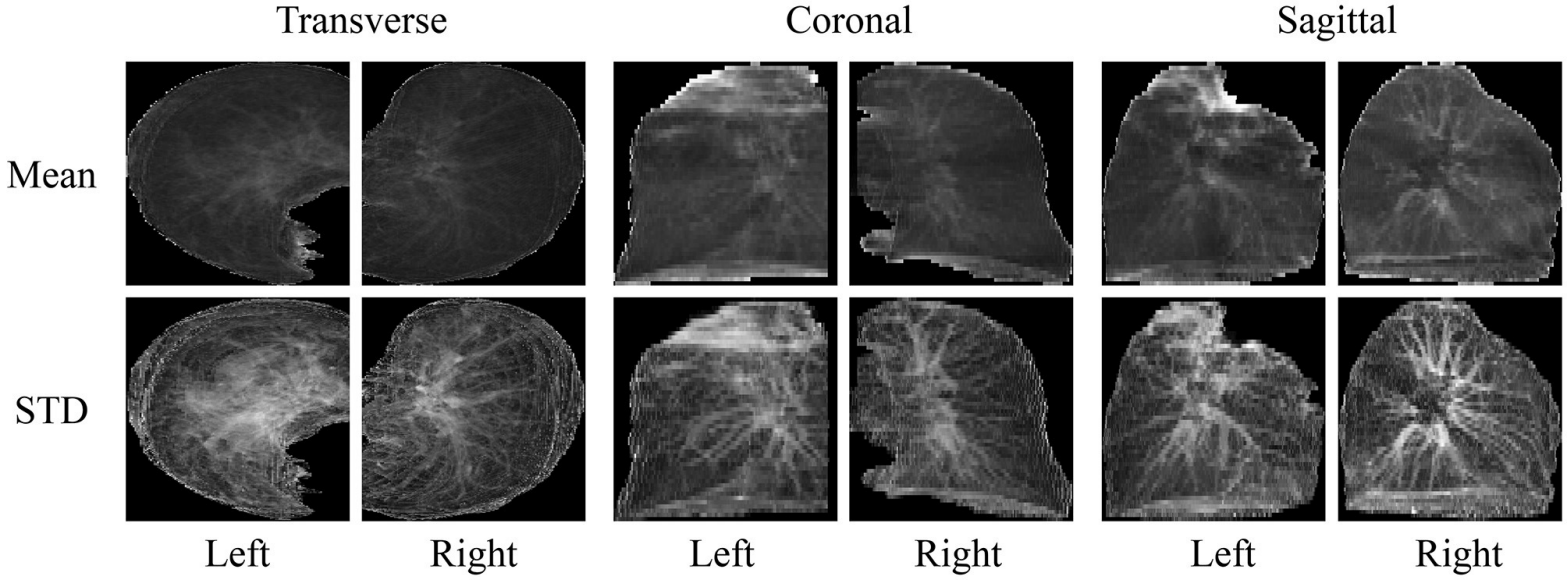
2D projection results generated from 3D masked CT.
